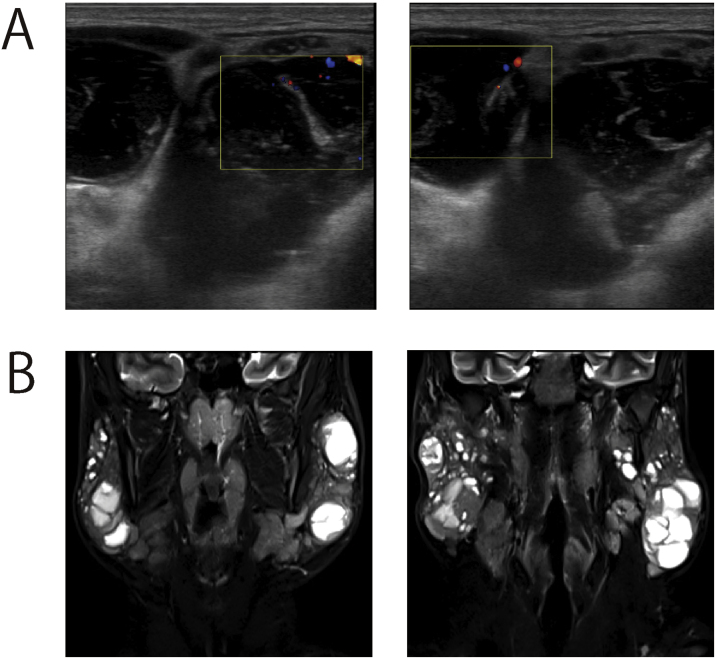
Imaging examination of a patient with benign lymphoepithelial cysts. (A) Ultrasound images. Multiple cystic lesions were detected in both parotid glands. (B) Numerous cystic lesions and no obvious tumors were observed on ultrasound imaging.
